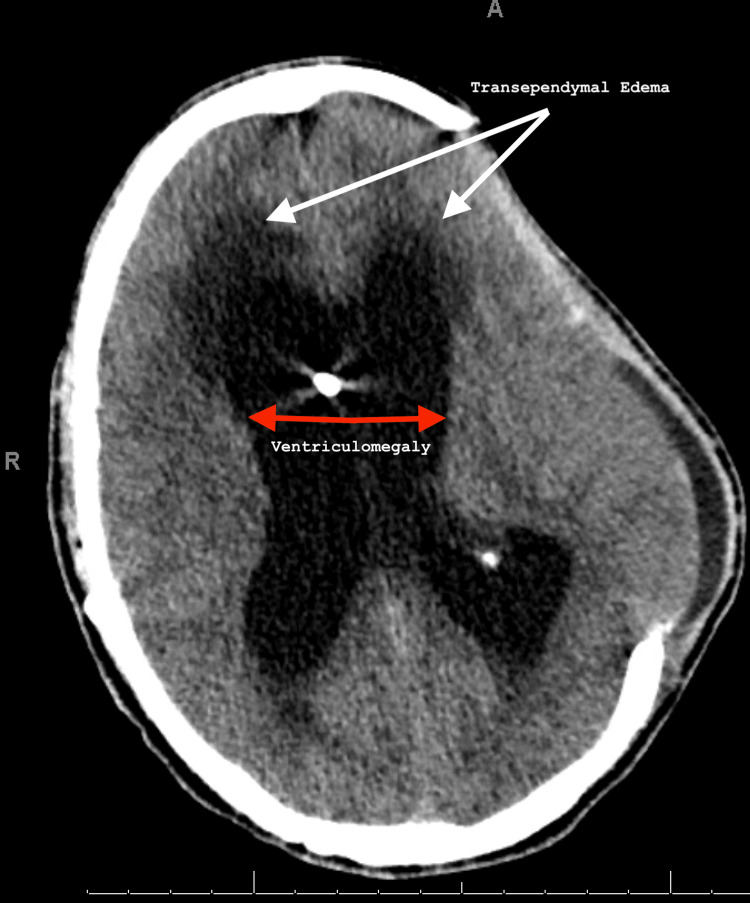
Axial contrast-enhanced CT brain demonstrating severe ventriculomegaly with enlargement of the lateral ventricles (red arrows) and surrounding periventricular hypodensity consistent with transependymal cerebrospinal fluid flow/edema (white arrows), concerning for ventriculoperitoneal shunt dysfunction. CT: computed tomography
